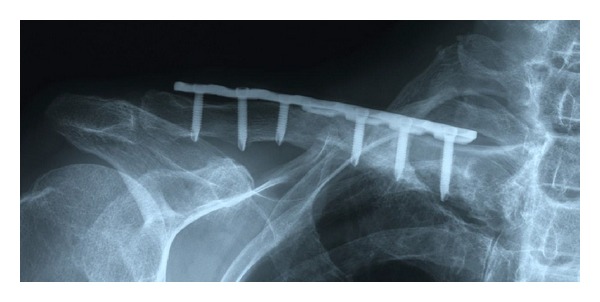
One-year postoperative radiograph demonstrating successful bony union.
